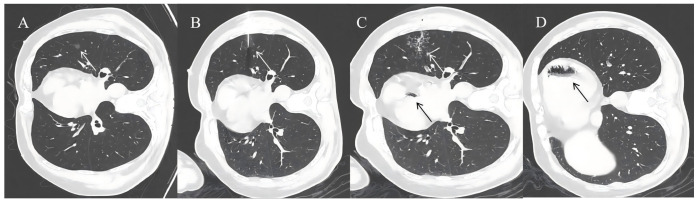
A 60-year-old male patient with no prior medical history. (A) Unenhanced chest CT revealed a pure ground-glass nodule (pGGN) in the anterior segment of the left upper lobe, measuring approximately 7.0 × 6.0 mm (white arrow). (B) Given that pGGNs are notoriously difficult to localize precisely under video-assisted thoracoscopic surgery (VATS), preoperative CT-guided placement of a metallic fiducial marker was indicated (white arrow). (C) Following marker deployment, ill-defined patchy opacification was observed surrounding the target lesion, consistent with procedural hemorrhage (white arrow), while intravascular gas was identified at the aortic root (black arrow). (D) CT image demonstrated the intravascular gas extending into the left pulmonary vein, the apex of the left ventricle, and its lateral wall (black arrow).
